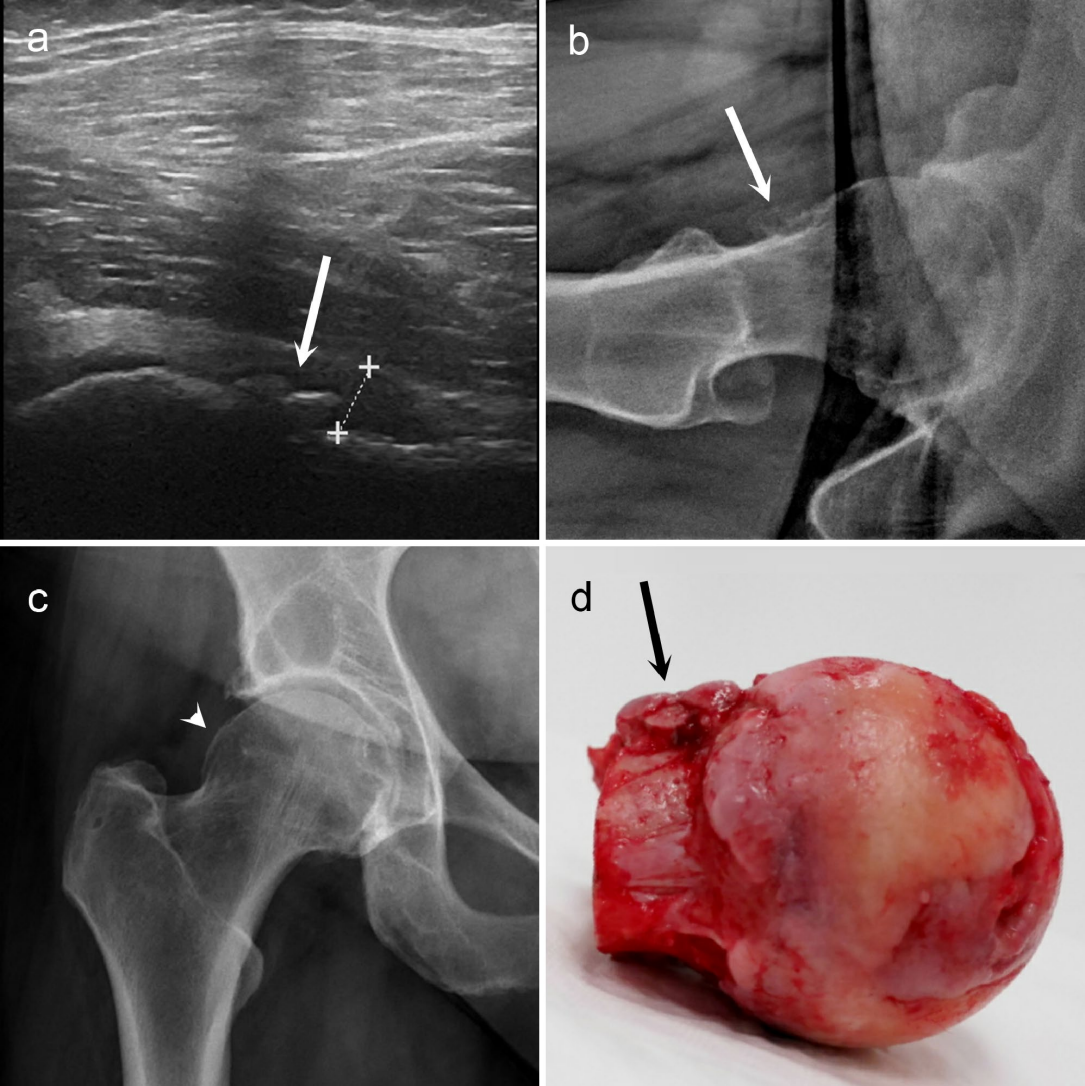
Large femoral collum osteophytes (white arrows) in longitudinal ultrasonographic view (a) and lateral conventional radiographic view (b). In the antero-posterior radiography (c), the same osteophyte is barely visible (white arrowhead). During the total hip arthroplasty, the femoral head was removed, and the corresponding osteophytes (black arrow) are clearly distinguishable at the femoral collum (d). In ultrasonography, a small effusion is also observed (measured within the dashed lines).
